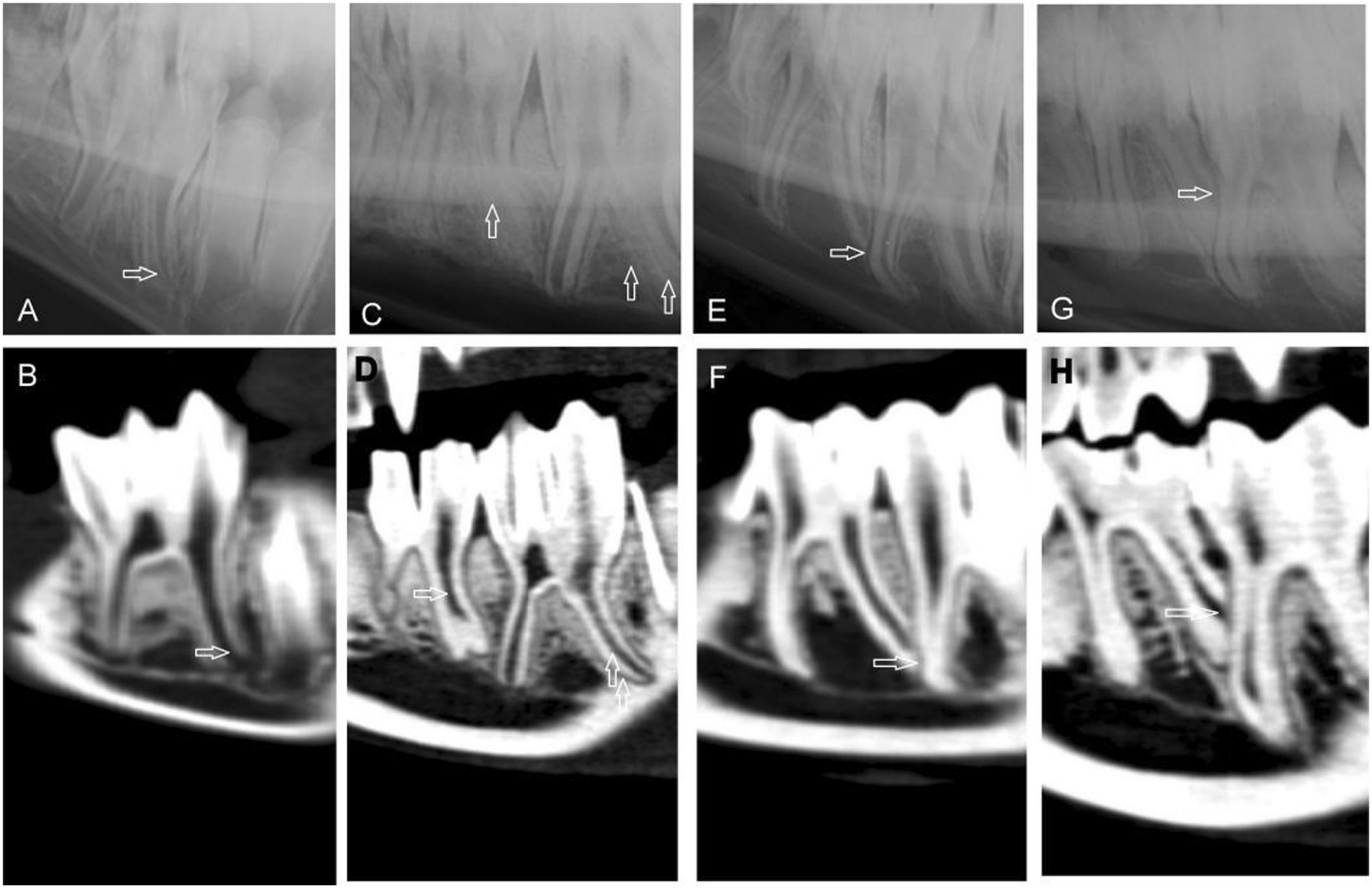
Findings in curved mandibular tooth roots at different ages. Digital radiographs (lateral30°ventral - laterodorsal oblique projection, upper row) and sagittal CT-reconstructions (lower row) of mandibular teeth showing distal root shape in different ages. A/B: Caudal deviation of the distal root of a deciduous 08 in a 9 month old alpaca. C/D: Sclerotic changes in the alveolar bone and curved shape of the distal roots of 409 and 410 in a 31 month old llama. E/F: Distal 09 root in contact with mesial root of 10, there was no alveolar bone between the two neighboring teeth in this 61 month old alpaca. G/H: Deformed distal root of 09 and exposed root canal cavity in a 107 month old llama. Described findings are indicated with white arrows.
